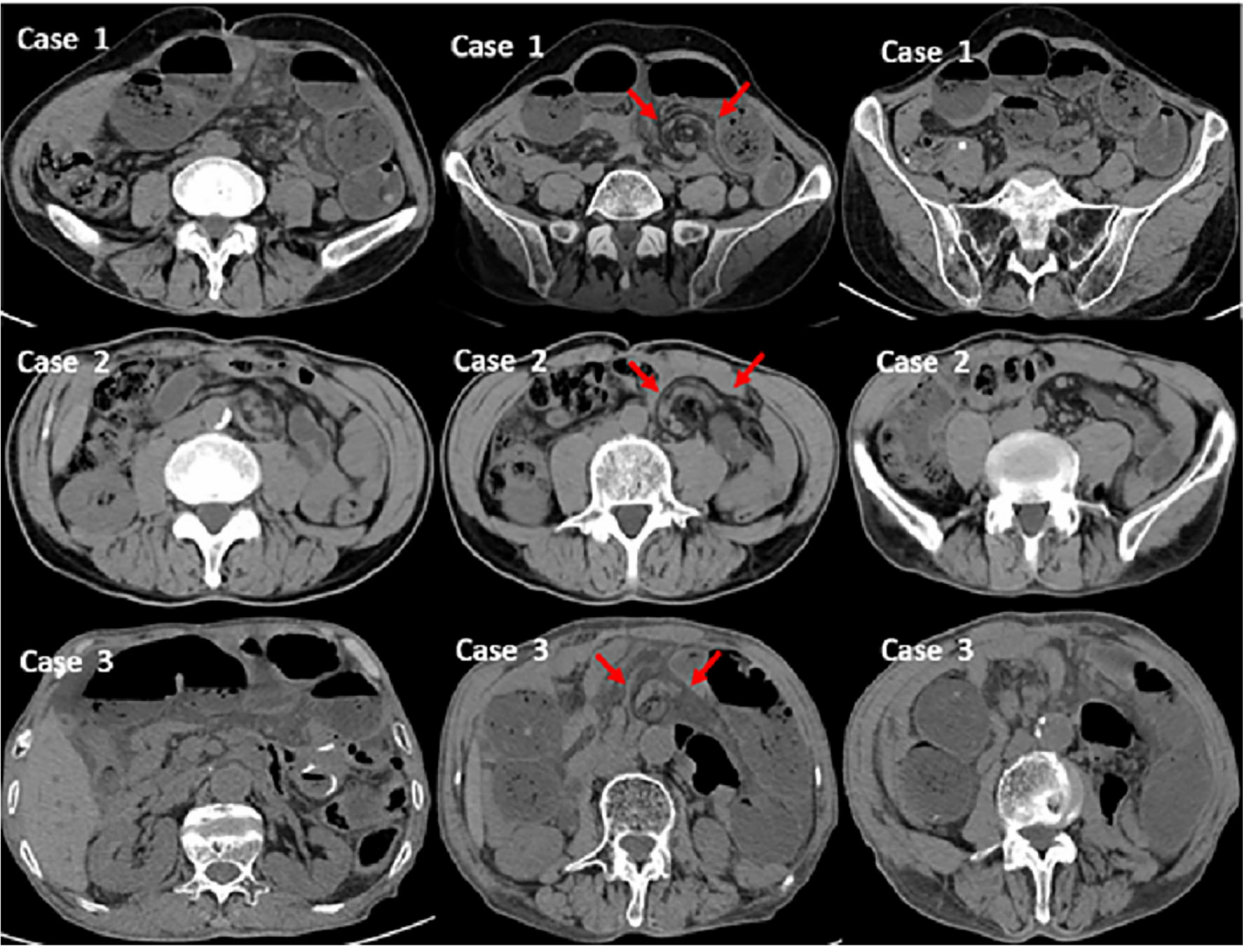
Whirling appearance of the mesentery and mesenteric vessels on CT scans (“whirl sign”, red arrows)
